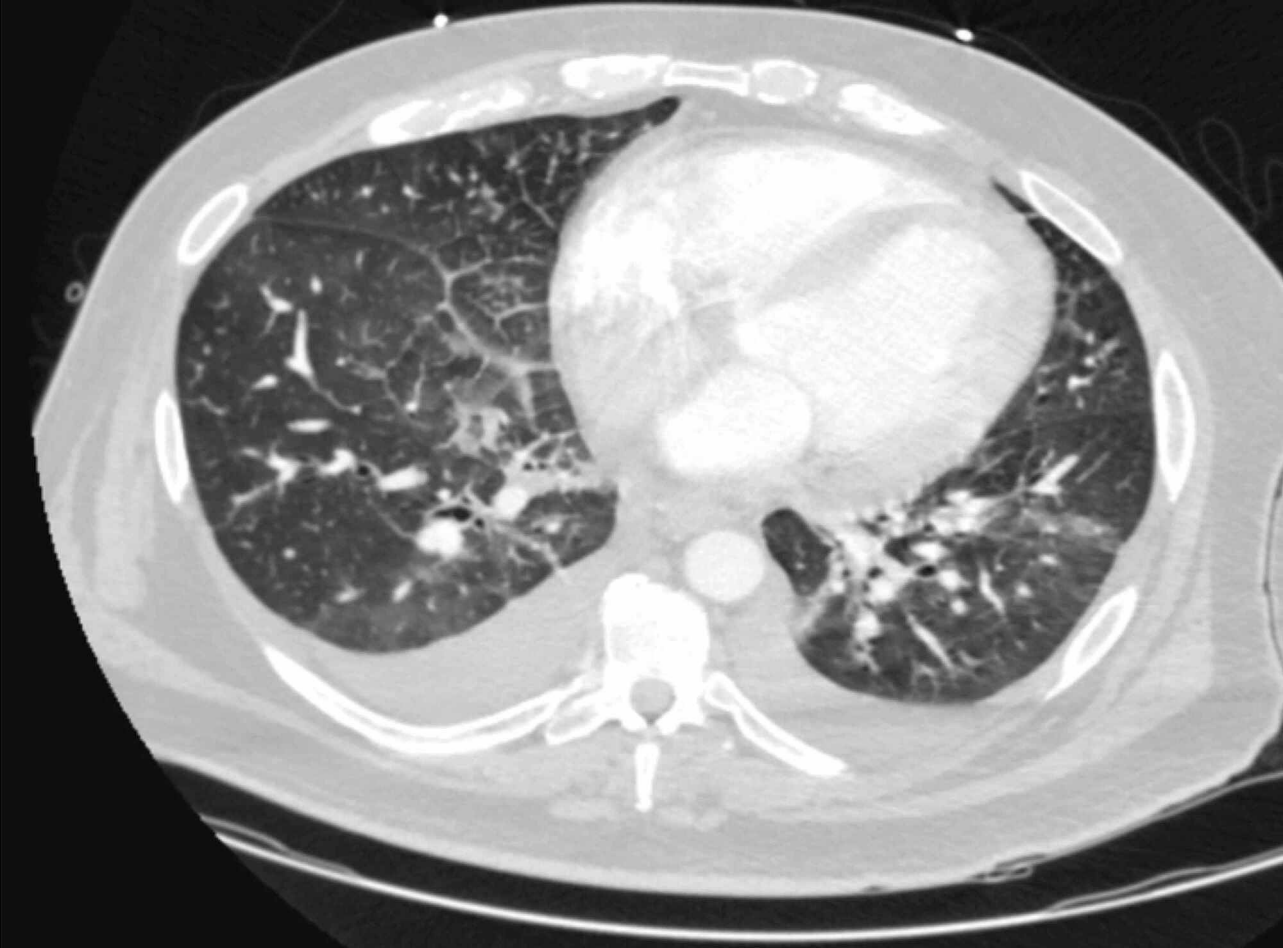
CT scan of the chest with contrast showing ground-glass opacities involving bilateral lower lobes
